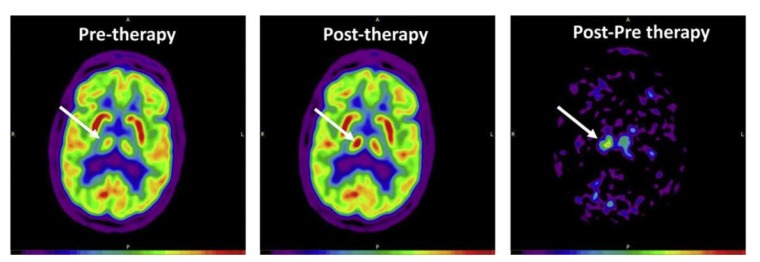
An example of FDG-PET image, taken from Rudroff et al. 2019 [25]. FDG-PET transaxial image acquired pre- and post-tDCS therapy. Images are scaled in standardized uptake values normalized to the global mean value (Max = 1.88 for pre-and post-therapy and 0.5 for post-pre therapy). The white arrow indicates the right thalamus, the area with the greatest difference between the images. The color bar describes increasing FDG uptake with increasing signal intensity (from black, indicating no glucose uptake, to red, indicating the greatest glucose uptake).
